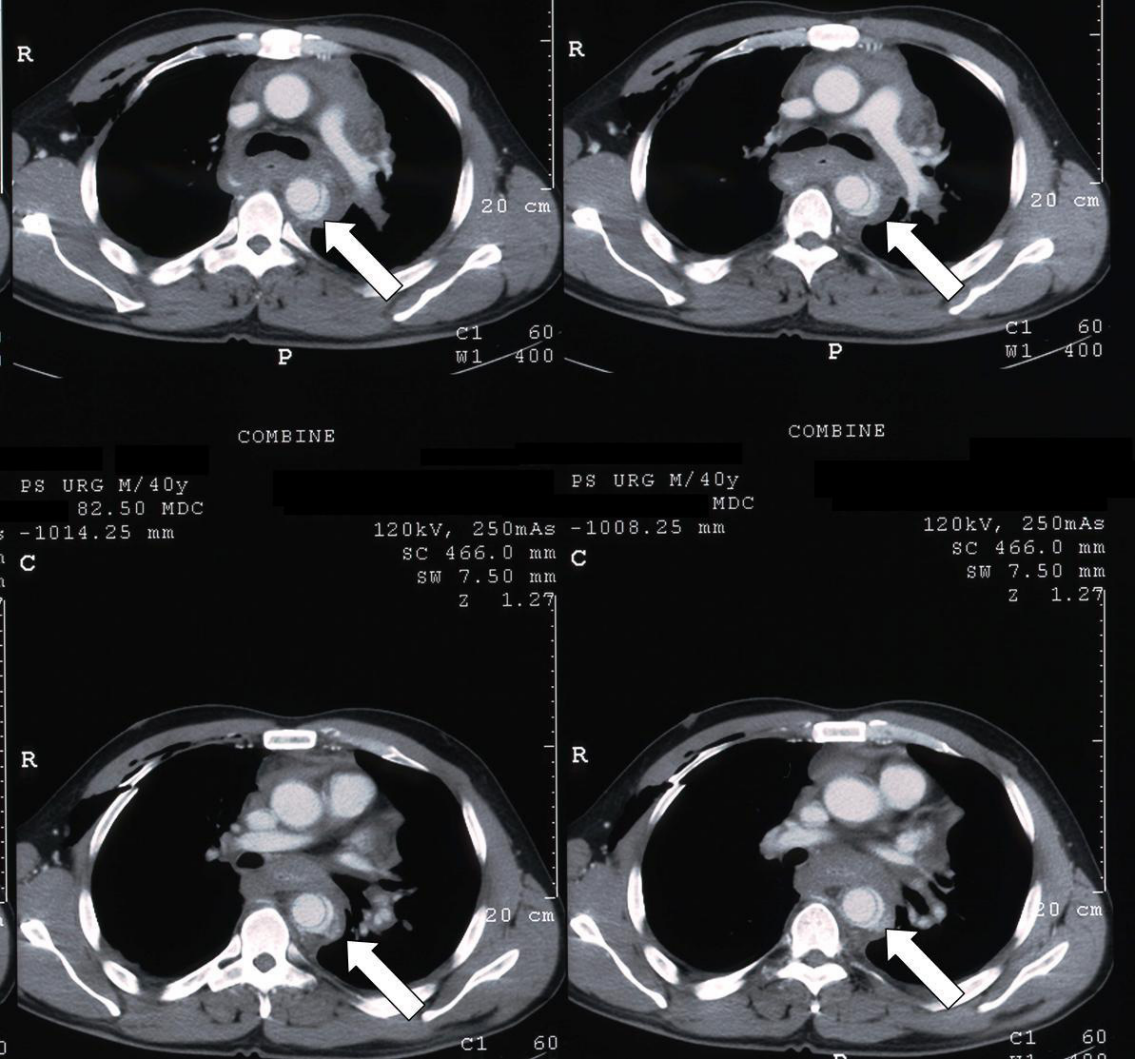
Pre-operative CT scan. Preoperative CT-scan showing isthmus rupture with dissection of the thoracic aorta.
